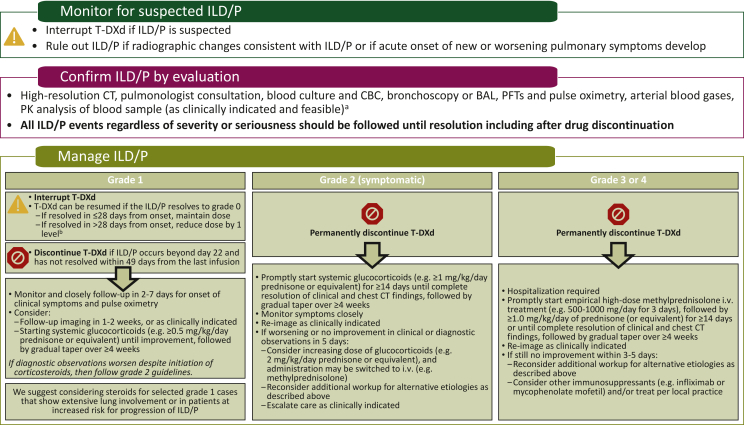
Management of ILD/pneumonitis.^20^^,^^59^^,^^61^^,^^62^ The ‘Manage ILD/P’ portion of this figure is from Figure 3 of Swain SM, Nishino M, Lancaster LH, et al. Multidisciplinary clinical guidance on trastuzumab deruxtecan (T-DXd)-related interstitial lung disease/pneumonitis-Focus on proactive monitoring, diagnosis, and management. *Cancer Treat Rev*. May 2022;106:102378.^62^https://doi.org/10.1016/j.ctrv.2022.102378, Creative Commons license and disclaimer available from https://creativecommons.org/licenses/by-nc-nd/4.0/. BAL, bronchoalveolar lavage; CBC, complete blood count; CT, computed tomography; ILD/P, interstitial lung disease/pneumonitis; i.v., intravenous; PFT, pulmonary function test; PK, pharmacokinetics; q3w, every 3 weeks; T-DXd, trastuzumab deruxtecan. ^a^Evaluations should include high-resolution CT, pulmonologist consultation (infectious disease consultation as clinically indicated), blood culture and CBC (other blood tests should be considered as needed), bronchoscopy and BAL if clinically indicated and feasible, PFTs and pulse oximetry, arterial blood gases if clinically indicated, and one blood sample for PK analysis as soon as ILD/P is suspected if feasible. ^b^See Table 3 for the dose reduction levels.
